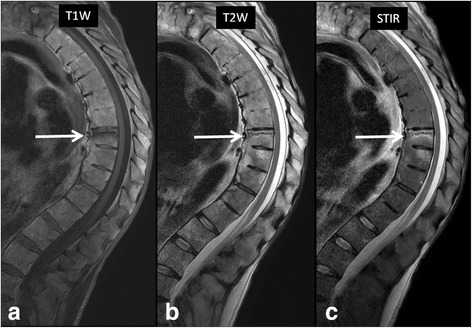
52-year-old man with known history of ankylosing spondylitis presented with acute back pain following a fall. a T1-weighted, b T2-weighted and c STIR images in the sagittal plane shows a horizontal fracture *line* with associated bone marrow edema involving the superior endplate of T8 vertebral body (*arrows*). The patient showed no clinical evidence of active infection
